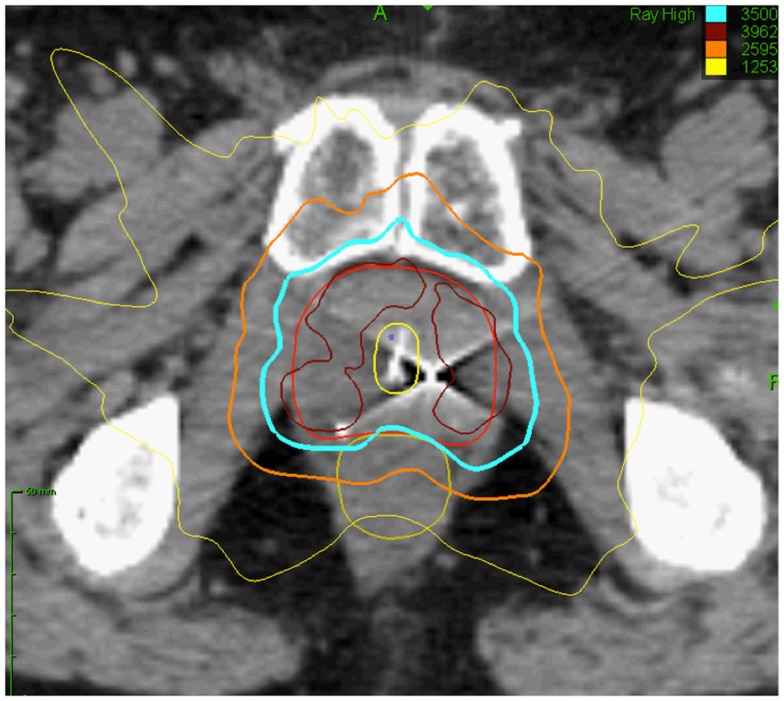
Prostate SBRT: treatment planning axial computed tomography images demonstrating the prostate (red line), prostatic urethra (yellow), and rectum (green line). Isodose lines shown as follows: 115% of the prescription dose, maroon line; 100% of the prescription dose, light blue line: 75% of the prescription dose, orange line; and 35% of the prescription dose, green line.
